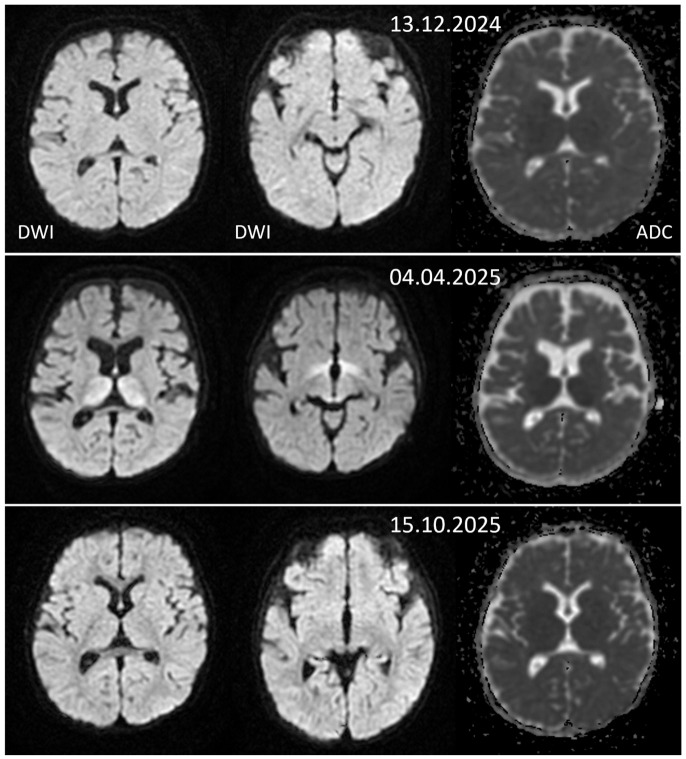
Brain MRI figures performed: at the age of 4 months (13 December 2024), at the age of 8 months (4 April 2025) and at the age of 14 mouths (15 October 2025). Comparison of MRI of DWI/ADC sequences—images from 4 April 2025 showed restricted water diffusion in both the thalami and the lower part of both internal capsules associated with cytotoxic edema in these areas. In the examination from 15 October 2025, these changes resolved. The examination was performed with a GEMS Artist 1.5T device. Abbreviations: MRI—Magnetic Resonance Imaging, DWI—Diffusion-Weighted Imaging, ADC—Apparent Diffusion Coefficient.
